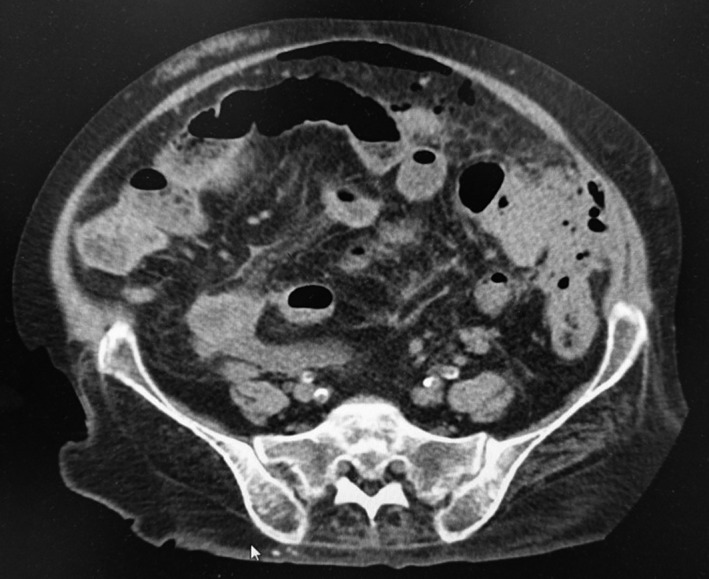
This is Hinchey stage III disease. Computed tomography image shows perforated diverticulitis of sigmoid colon with free air that caused generalized peritonitis. A two‐stage procedure, Hartmann's procedure was carried out
